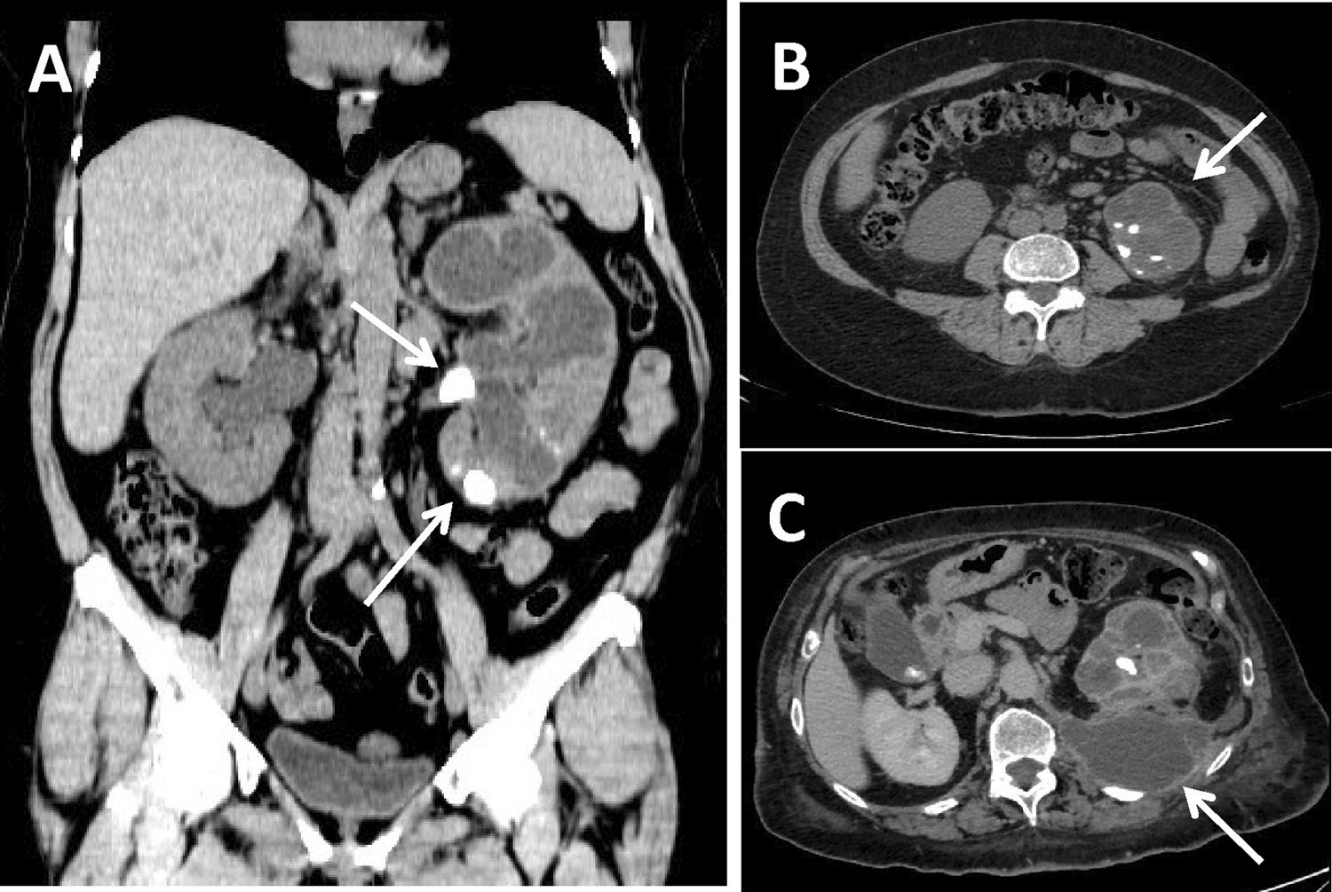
CT staging of xanthogranulomatous pyelonephritis. a A 76-year-old female patient presenting with mild fever and stage I XGP with multiple voluminous high-density staghorn calculi in the contracted renal pelvis and inferior calyxes (arrows), concomitant calyx dilatation and cortical thinning. b A 68-year-old male patient presenting with weight loss and mild fever and stage II XGP where, besides the presence of multiple hypodense areas of xanthogranulomatous tissue inside an inhomogeneous parenchyma, is evident a thickening of the Gerota fascia with streaks of perirenal fat (arrow) and enlarged lymph nodes at the renal hilum and in the para-aortic region. c A 82-year-old female patient presenting with pain, fever and hematuria and severe stage III XGP with a voluminous fluid collection bounded by vascularized tissue in the retroperitoneal space involving the psoas muscle (arrow)
